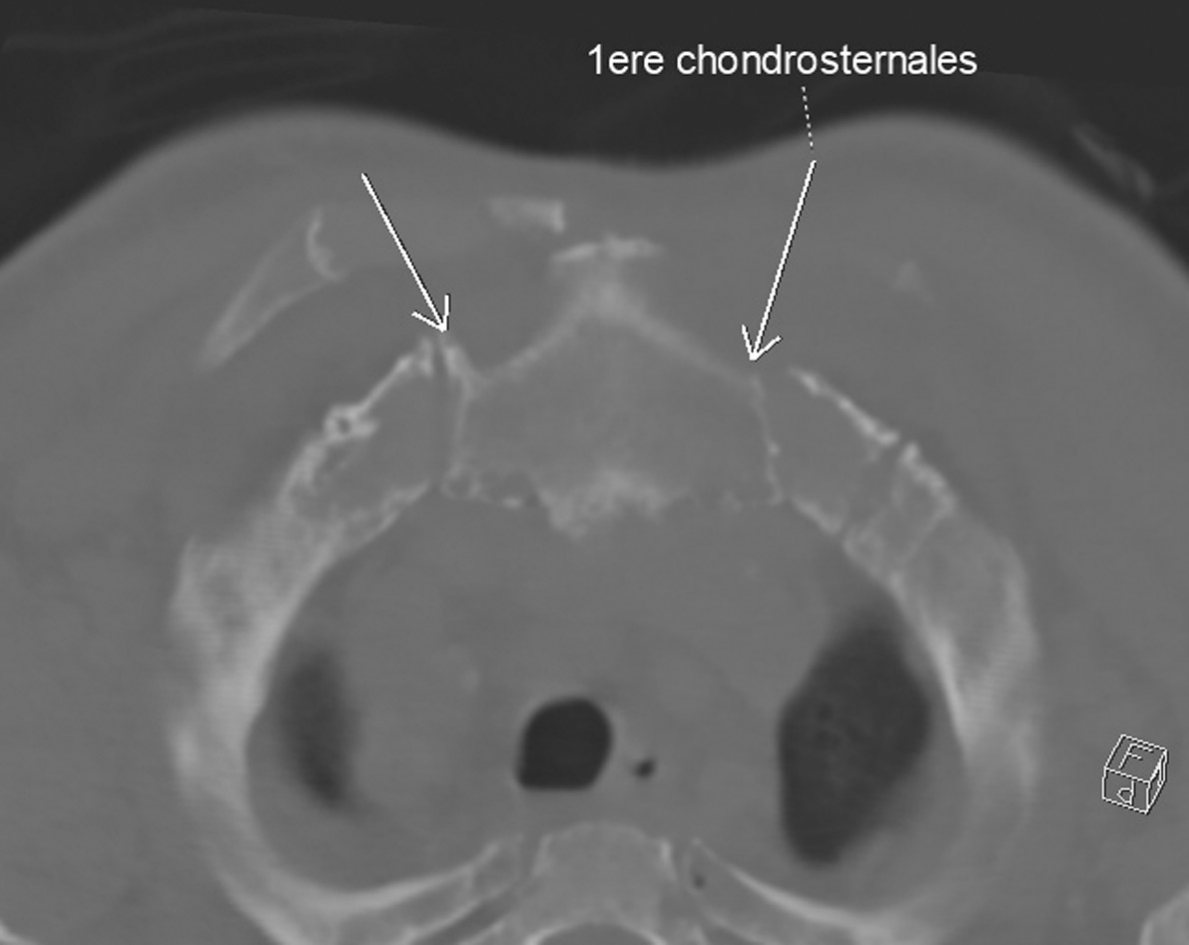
Multifocal amyloid arthropathy in the sternoclavicular joints in CT scan
